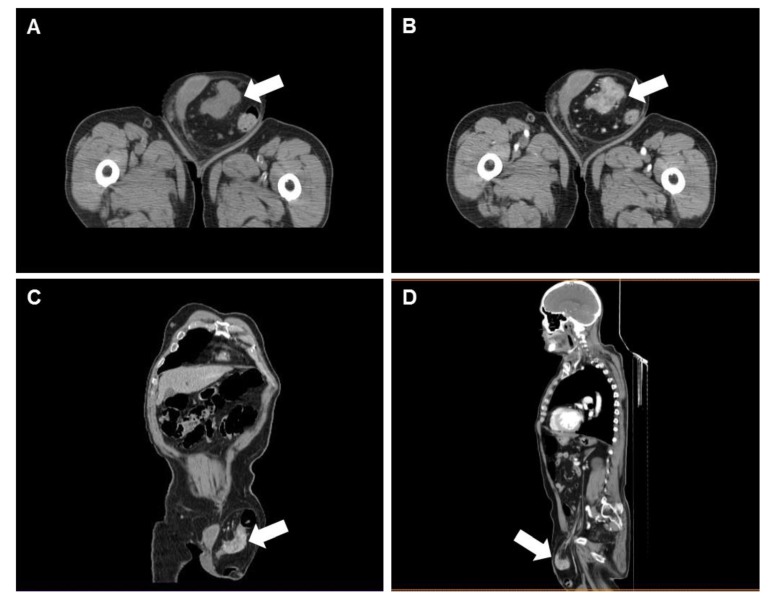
Computed tomography (CT) scan of sigmoid colon carcinoma incarcerated in inguinal hernia.: (a) axial plane without contrast injection; (b) axial plane with contrast enhancement; (c) coronal plane with contrast; (d) sagittal plane with contrast. Note: The arrows identify the pathological concentric thickening of the sigmoid wall in four different views
